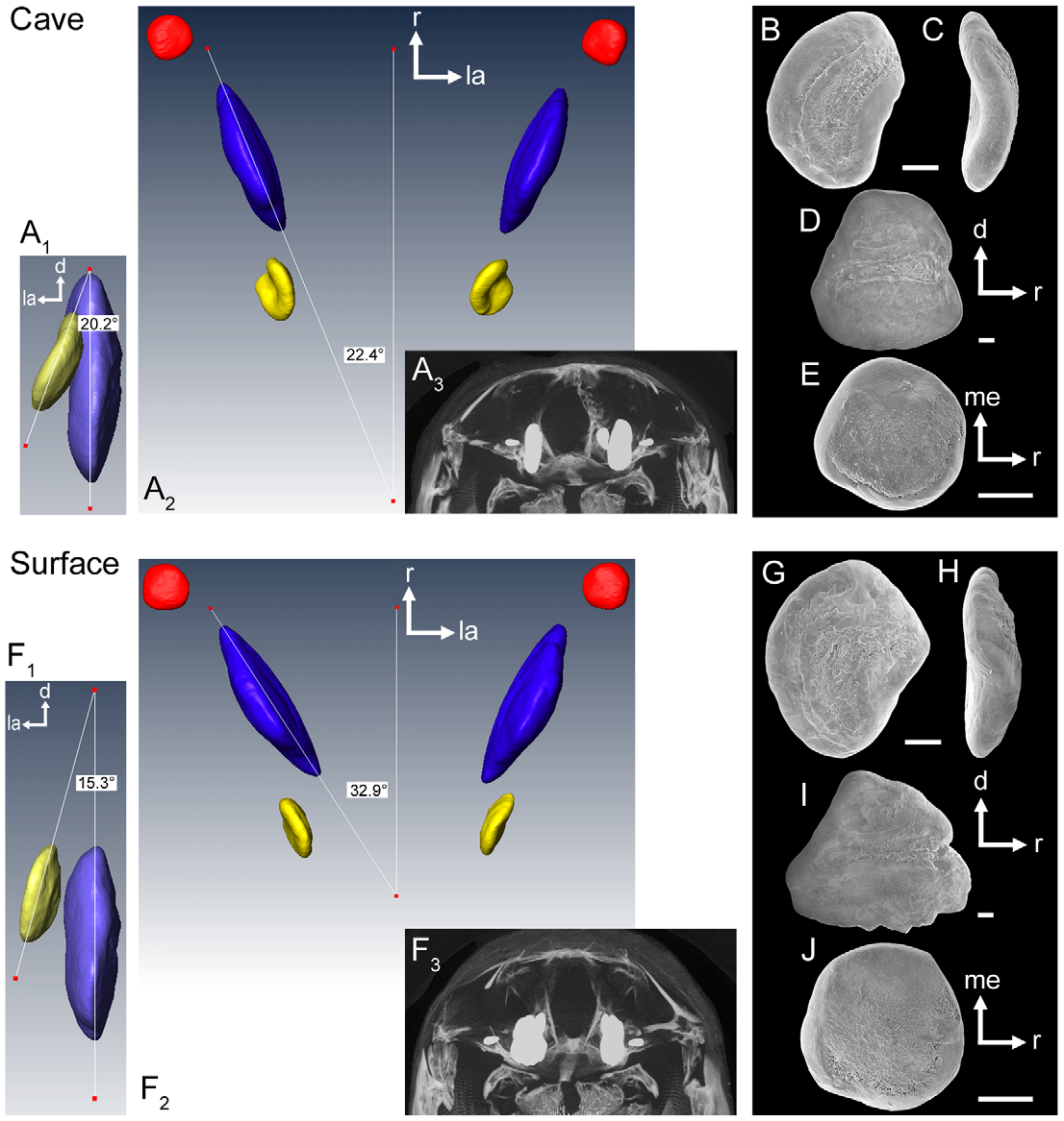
Position of otoliths *in situ*. Three-dimensionally reconstructed otoliths from a cave (SL = 35 mm) and a surface fish (SL = 38 mm, both females) based on μ-CT analyses. In (A_1_) and (F_1_) the left asteriscus and sagitta are shown in caudal view. (A_2_) and (F_2_): dorsal view of left and right lapilli (red), sagittae (blue), and asterisci (yellow). (A_3_) and (F_3_): brightest point projection of μ-CT sections of the neurocranium and otoliths *in situ* shown in rostral view. (B–E) and (G–J): SEM images of the left otoliths of the cave and the surface fish in medial (B, D; G, I), rostral (C, H), and ventral views (E, J), respectively. B–C, G–H, asterisci; D, I, sagittae; E, J, lapilli. d, dorsal; la, lateral; me, medial; r, rostral. Scale bars = 100 µm.
